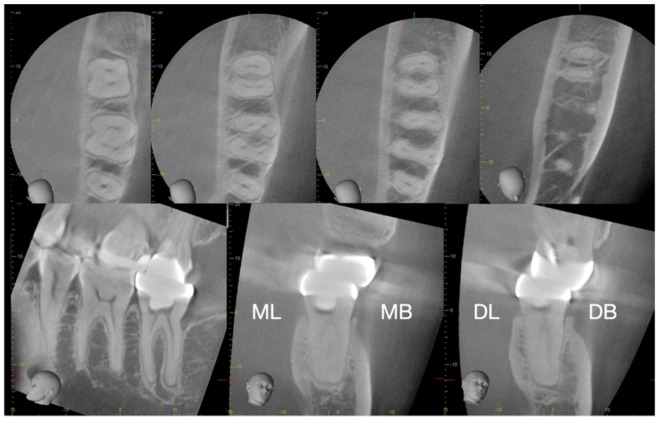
CBCT analysis of a mandibular left second molar. The cross-sectional images highlight how the canal orifices are obscured by endodontic triangles in both the mesiodistal and buccolingual dimensions. Such findings underscore the necessity of three-dimensional imaging to recognize constraints that would otherwise be invisible on standard periapical radiographs.
